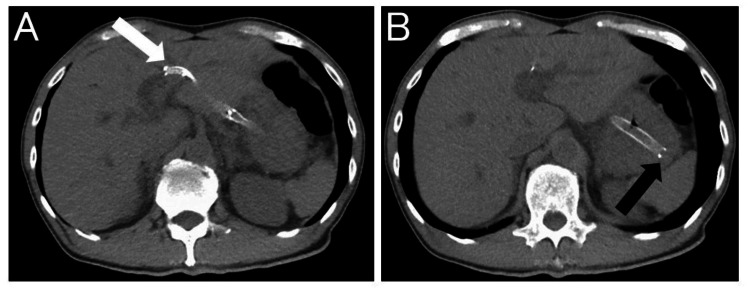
A CT scan on the next day showed no displacement of the placed stent. A: The tip of the stent (uncovered side) is located within the intrahepatic bile duct (white arrow). B: The posterior end of the stent is located within the stomach (black arrow).
